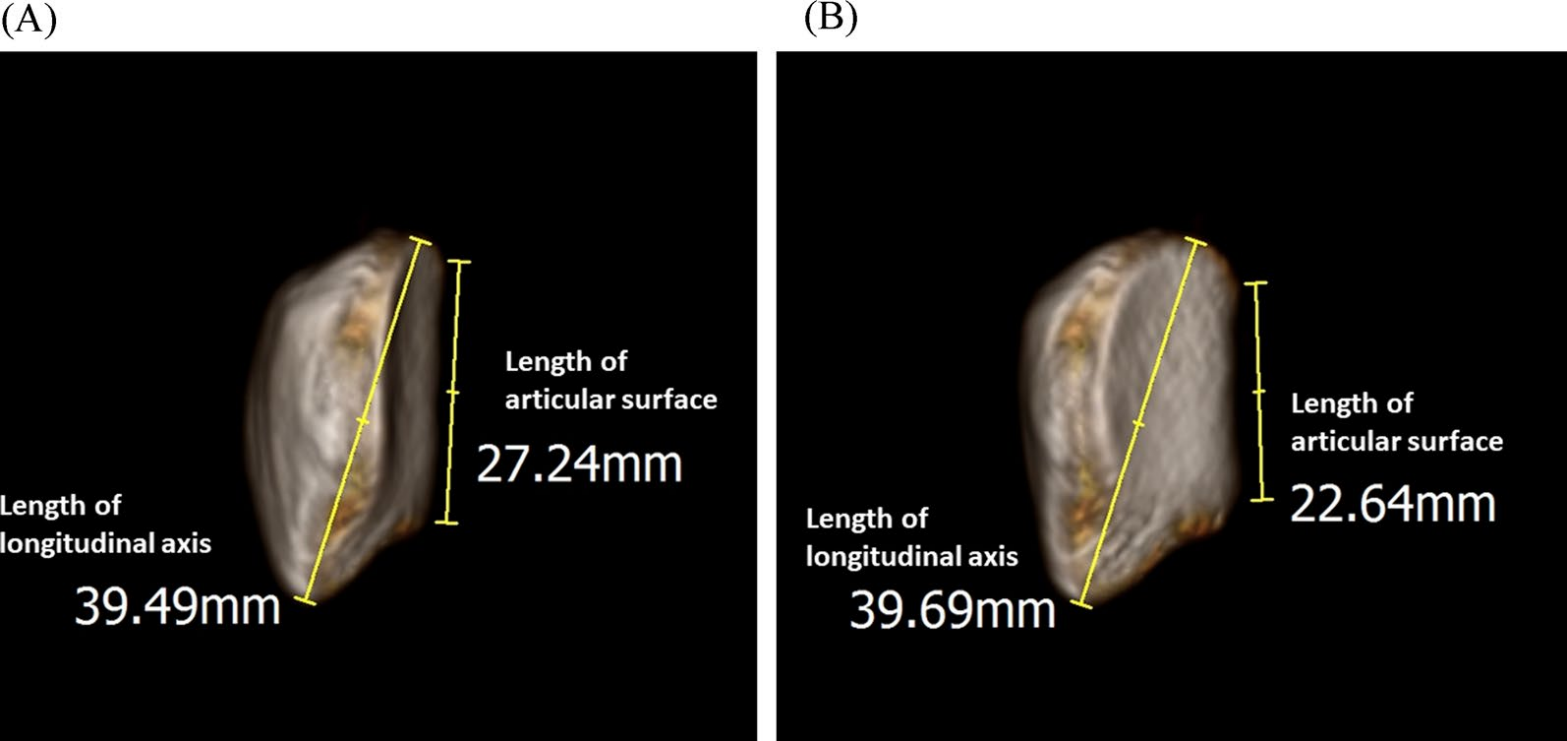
Length of the patellar articular surface and the longitudinal axis shown in the 2-dimensional sagittal angle. A Patella with no tilt.at 0° of knee flexion. B Patella with a medial tilt 5°at 30° of knee flexion. When the patellar tilt changes at 30° of knee flexion, the length of the articular surface shown in the 2-dimensional sagittal plane of the lateral radiograph can appear different from that at 0° flexion. However, the length of the longitudinal axis connecting the superior and inferior poles of the patella remains relatively constant in the sagittal plane, regardless of tilt
